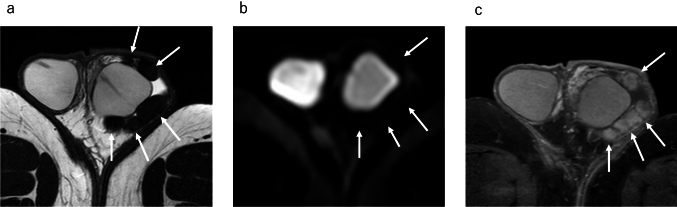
Fibrous pseudotumor in a 28-year-old male with left paratesticular masses. a Axial T2-weighted MR image shows marked hypointense multiple masses (white arrows), which are in contact with tunica vaginalis. b Axial diffusion weighted MR image shows masses (white arrows) with marked low-signal intensity. It is difficult to identify the location of masses without referring to other sequencing images. c Axial contrast-enhanced fat-suppressed T1-weighted MR image shows heterogeneous enhancement of the masses (white arrows)
